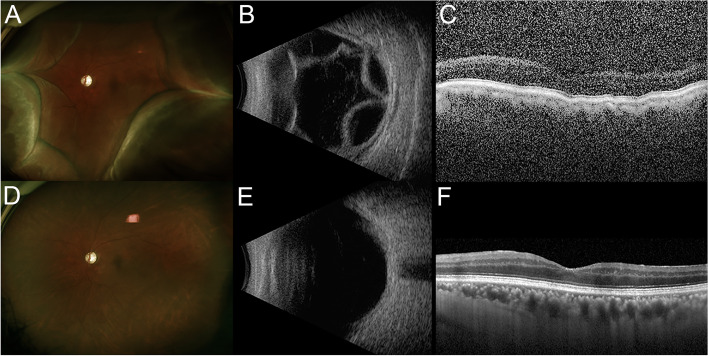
A-C Choroidal detachment 21 days after selective laser trabeculoplasty (SLT). A Wide-field fundus photography and (B) B-scan ultrasonography showing choroidal detachment, accompanied by an intraocular pressure of 4 mmHg. C Optical coherence tomography (OCT) demonstrating a chorioretinal fold due to hypotonic maculopathy. D-F Improvement of choroidal detachment 2 months after SLT. D Wide-fundus photography and (F) OCT showing a flat retina without folding. E B-scan ultrasonography showing complete resolution of choroidal effusions
